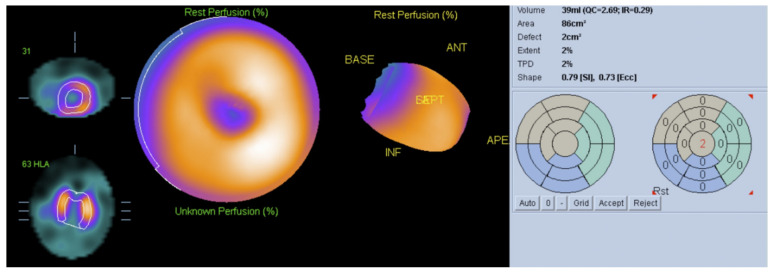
Automatic quantification (polar map) from rest 99m-Technetium sestamibi scintigraphy demonstrating reduced myocardial perfusion in apical region in a patient with a small aneurism of the left ventricular apex.
